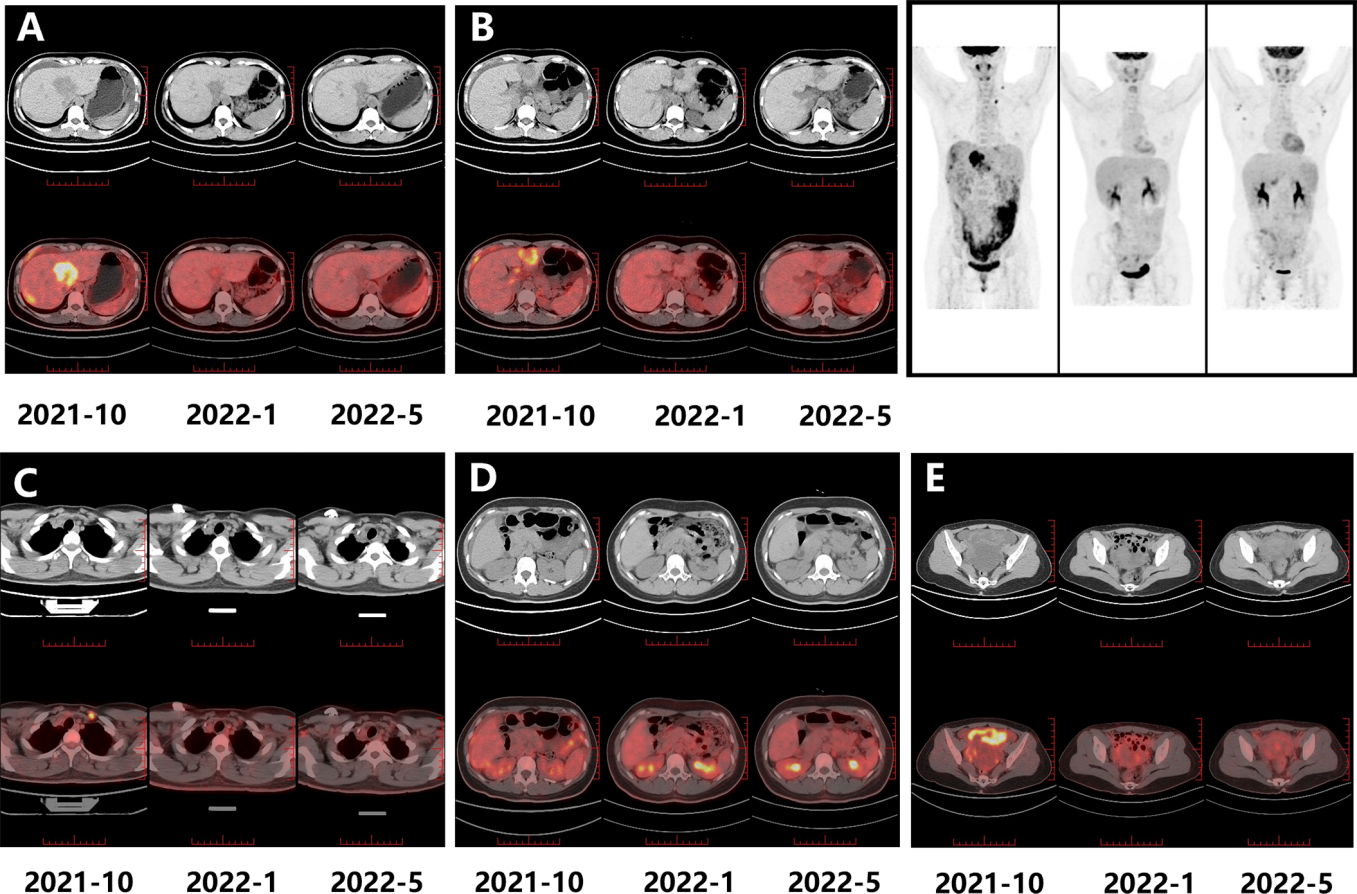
The PET-CT images for evaluating the treatment response. The metabolic activity change is noticeable in the masses located in the right liver (A), left liver (B), left cervical lymph node (C), retroperitoneal nodes (D), and omentum and ovary (E) (2021-10 is the baseline).
